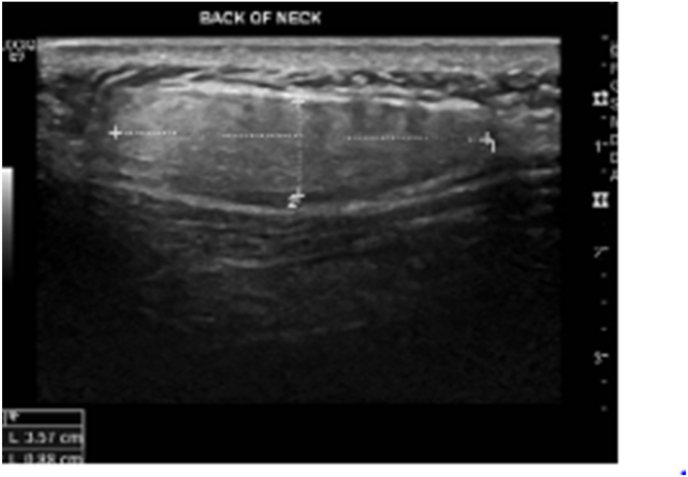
Hypoechoic mass in ultrasound imaging, on the back of the neck.
